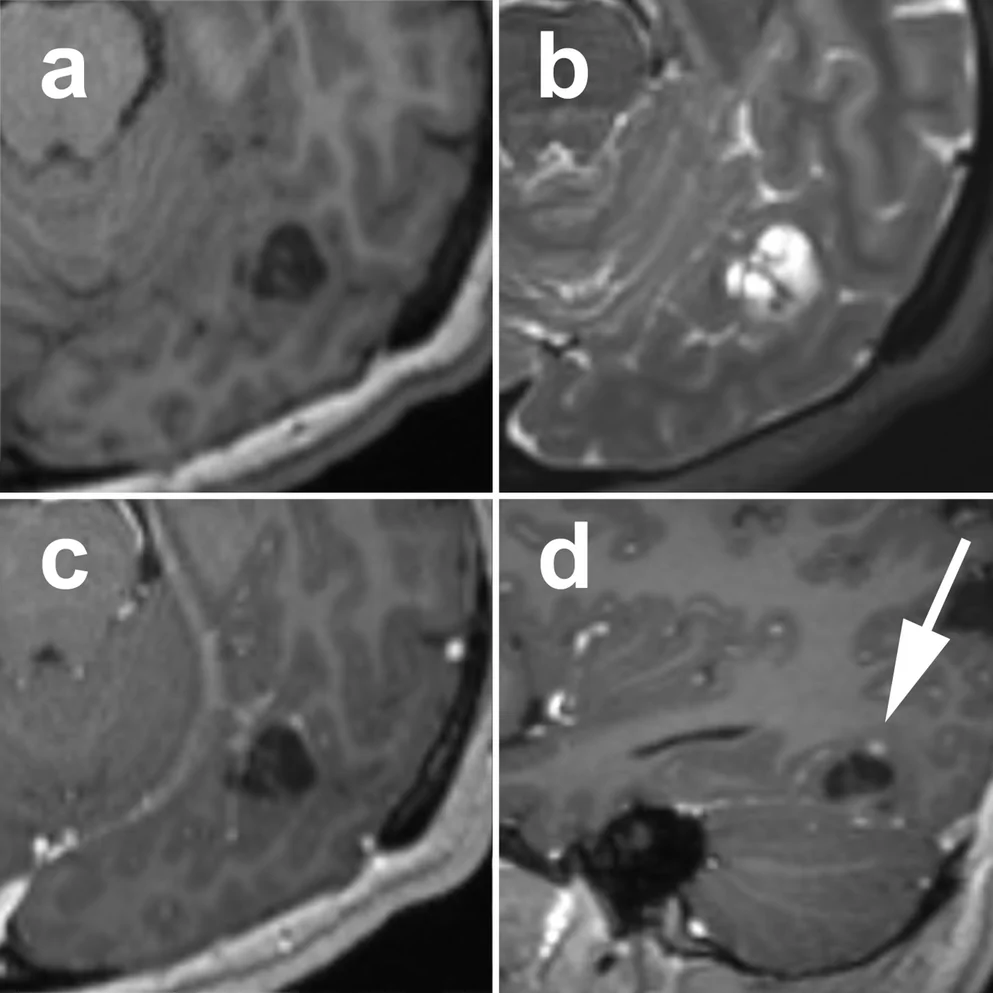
A 21-year-old woman with pleomorphic xanthoastrocytoma presenting with seizures. Axial T1-weighted image (a), axial T2-weighted image (b), axial and sagittal contrast-enhanced T1-weighted images (c and d, respectively). A cystic lesion with an enhancing mural nodule (arrow) is seen in the cortical region of the posterior lobe. No peritumoral edema is observed
